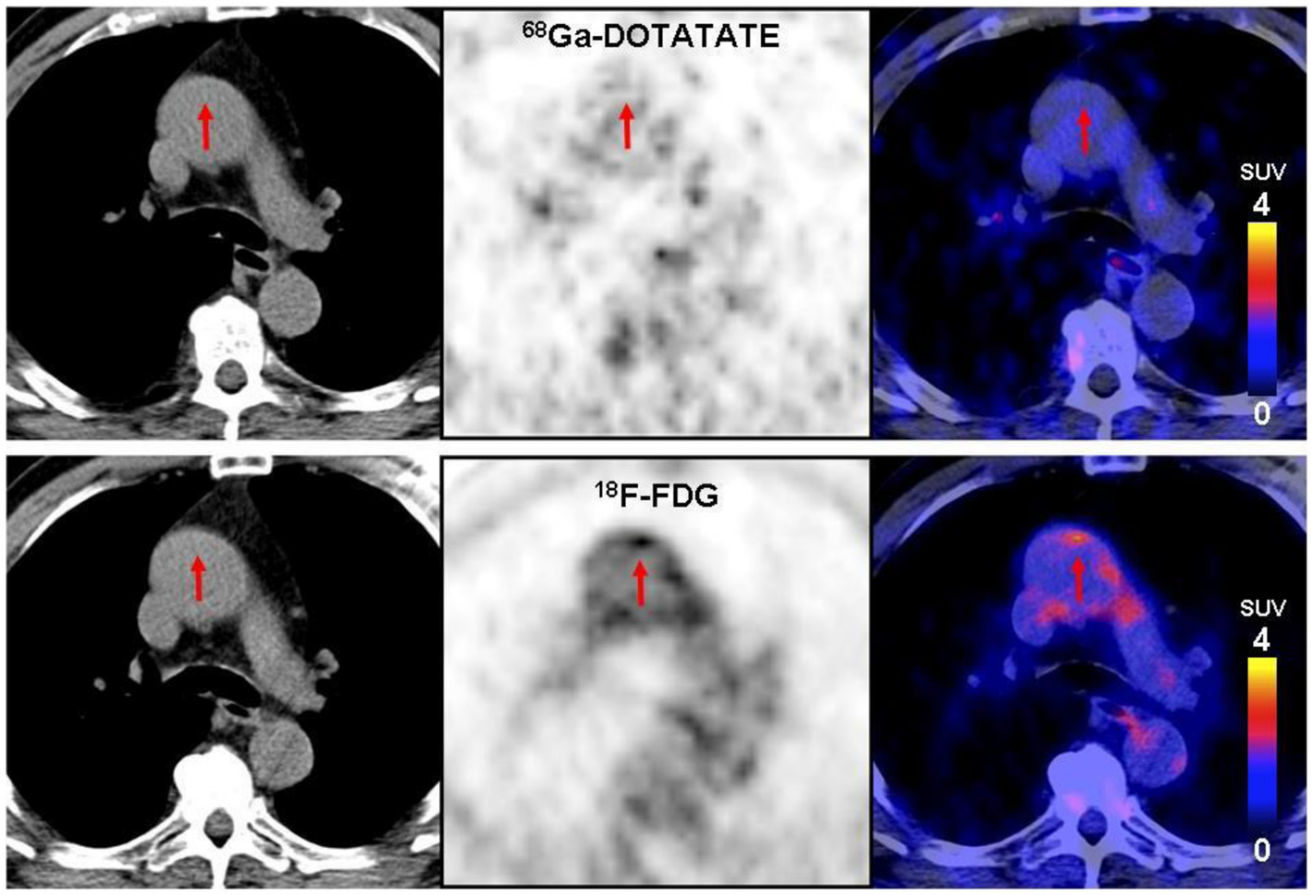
Focal uptake of FDG but not DOTATATE in the vessel wall. Transverse PET/CT images of a 77-year-old male patient. With hypertension, hypercholesterolemia, history of coronary disease and a family history of cardiovascular disease. Intense ^18^F-FDG uptake can be observed in the aortic arch (upper row), whereas no focally increased ^68^Ga-DOTATATE uptake can be seen (lower row). TBR_DOTATATE_ was 1.64, while TRB_FDG_ was 2.50.
